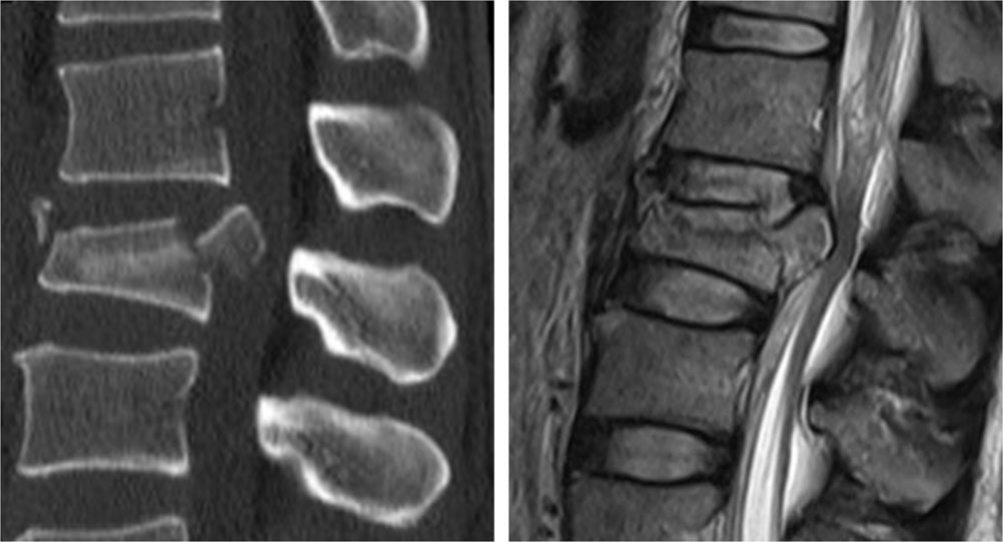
36-year-old man with an A2.2 fracture at level L1. T2WI magnetic resonance image shows that both the upper and the lower intervertebral discs are injured at a Grade 3 level.
